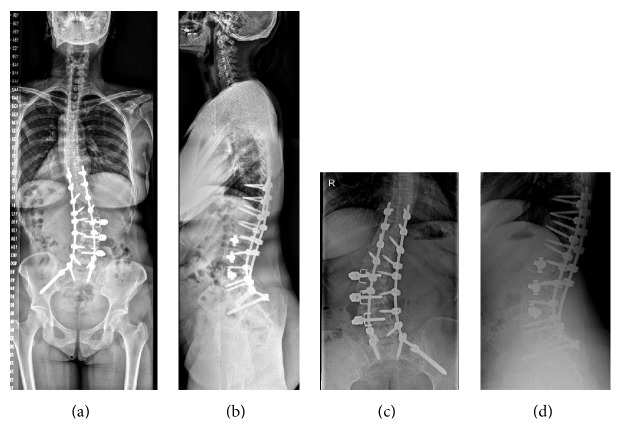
(a-b) Anterior and lateral spinal views with definitive instrumentation T-10 to iliac bone. (c-d) Lumbar X-ray views.
